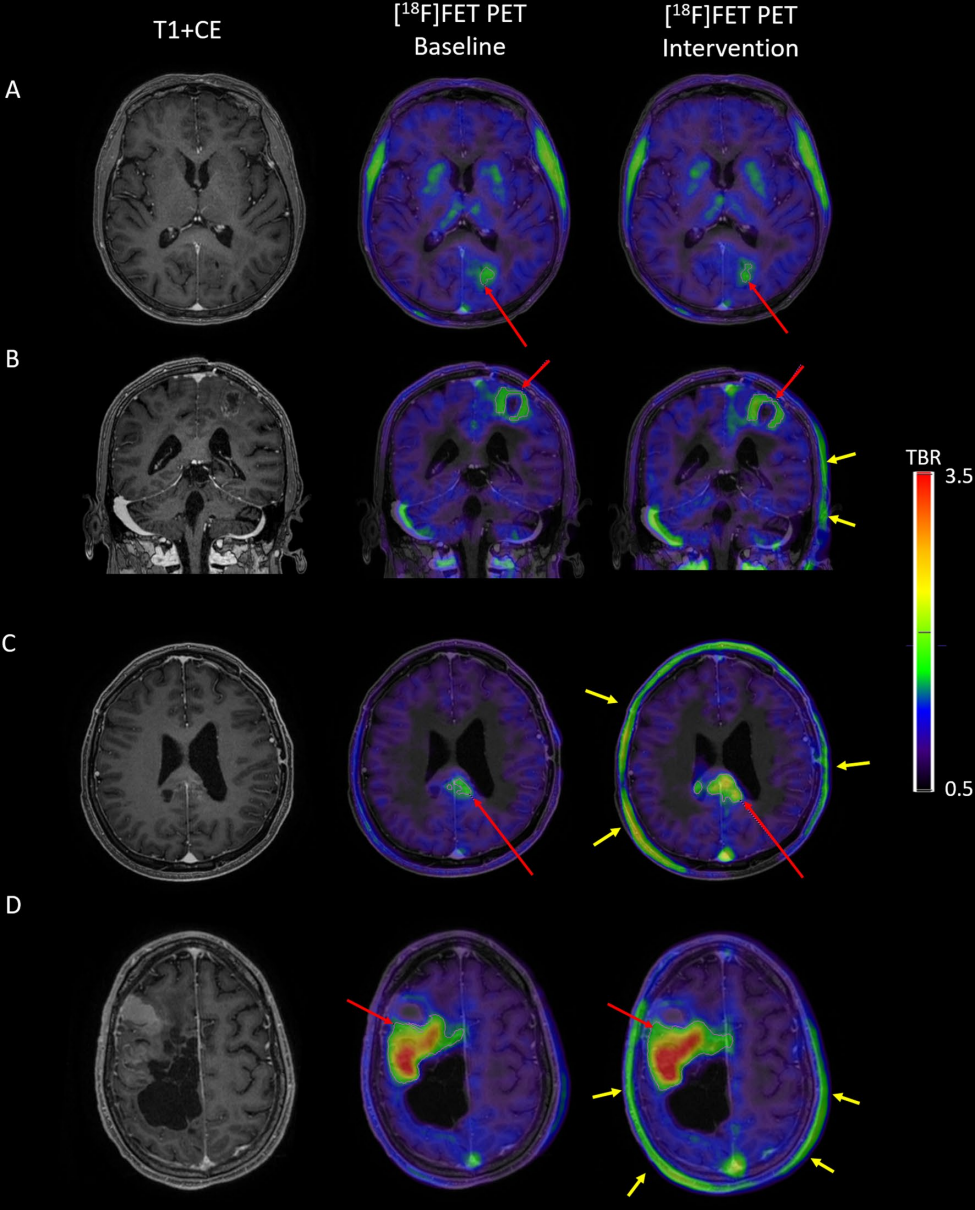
The influence of protein intake on [^18^F]FET PET uptake in tumour and extracerebral tissue. Left to right: post contrast T1 weighted MRI, baseline [^18^F]FET PET and intervention [^18^F]FET PET. Images are normalized to mean activity of a cortical background region. Repeat [^18^F]FET PET without oral protein intervention is shown in panel A and [^18^F]FET PET with and without oral protein intervention are shown in panel B-D. Note markedly relatively higher uptake in extracranial soft-tissue (yellow arrows), and also higher vascular activity in the post–protein intervention scans. A Patient #11, a 68-year-old female with oligodendroglioma, WHO grade 2. Baseline [^18^F]FET PET showed mild uptake in the left occipital region indicating active residual tumour with TBR_max_ of 1.75 and BTV of 1.06 ml. Seven days later a fasting intervention [^18^F]FET PET showed stable metrics (TBR_max_ 1.86 and BTV 0.66 ml). B Patient #2, a 65-year-old male with glioblastoma, WHO grade 4. Baseline [^18^F]FET PET showed mild-to-moderate uptake in left posterior frontal region with TBR_max_ of 1.99 and BTV of 8.0 ml. The protein intervention scan after consumption of 24 g of protein three days prior showed only slightly higher TBR_max_ of 2.18 and BTV of 9.2 ml. C Patient #1, a 56-year-old male with glioblastoma, WHO grade 4. Baseline [^18^F]FET PET showed mild uptake in splenium with TBR_max_ of 1.99 and BTV of 1.0 ml. The protein intervention scan after consumption of 48 g of protein three days later showed markedly higher TBR_max_ of 2.70 and BTV of 7.6 ml. D [^18^F]FET-PET with and without oral protein intervention: Patient #5, a 42-year-old male with astrocytoma, IDH mutant, WHO grade 3. [^18^F]FET PET showed uptake in the right frontoparietal region (TBR_max_ 4.56 and BT 34.4 ml). For the intervention scan seven days later scan 24 g of protein was consumed yielding an increase in TBR_max_ to 4.69 and BTV to 47.3 ml. The patient died two weeks after the intervention scan and increasing volume could reflect both protein-intake and tumour growth
